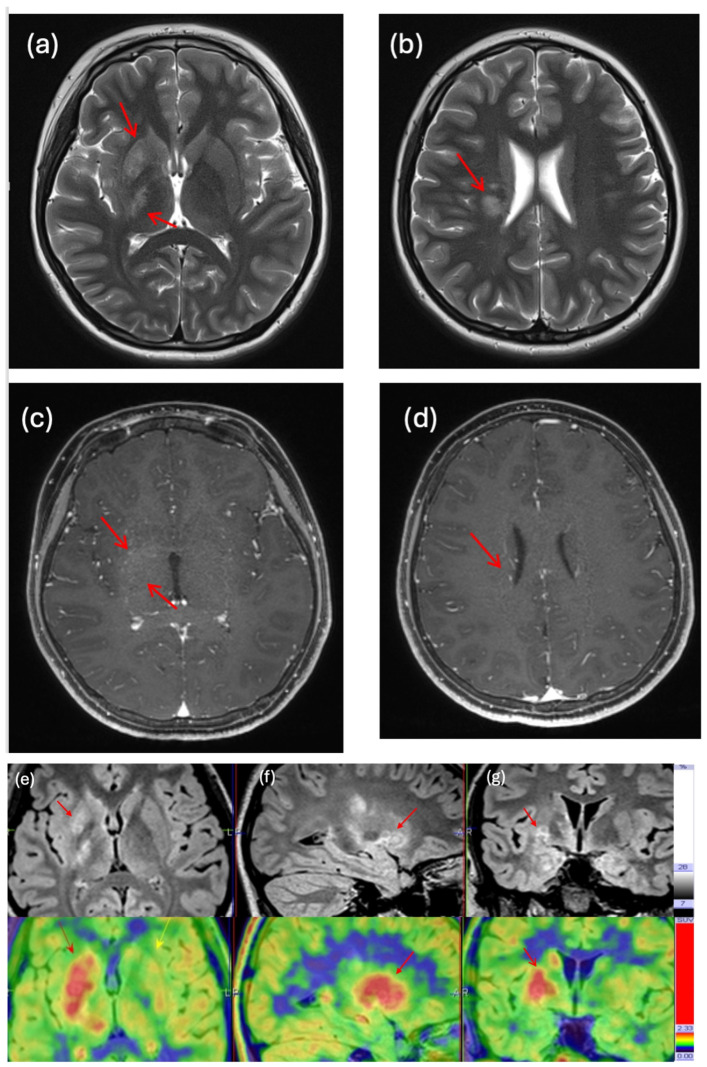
An 11-year-old boy (No. 3) presented with progressive left hemiparesis, initially diagnosed as ischemic stroke on the first MRI. PET-MRI helped to make the diagnosis of basal ganglia germinoma. (a,b) MRI showing hyperintense T2-weighted signal change at right basal ganglia extending to corona radiata with minimal mass effect on T2-weighted axial sequence (arrow). (c,d) MRI showing no significant enhancement along the whole-signal abnormality on post-gadolinium T1-weighted sequence (arrow). (e) Axial view, yellow arrow indicating normal contralateral side of the basal ganglia. (f) sagittal view, (g) coronal view of MET PET-MRI of the same patient showed a hyperintense T2-weighted/FLAIR signal at the right basal ganglia to corona radiata (upper row, red arrows). It demonstrated strong C11-methionine tracer uptake on PET-MRI (lower row, red arrows).
